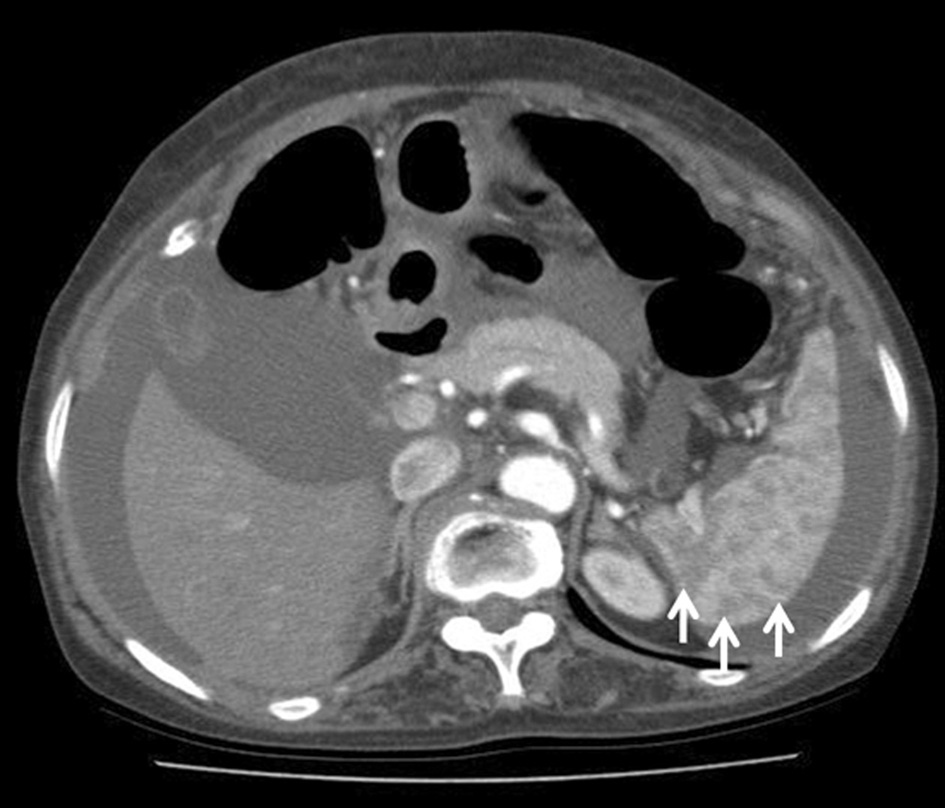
Initial abdominopelvic computed tomography reveals the spleen is occupied with multiple, well-defined, low density, lesions measured 0.5 to 1.5 cm in sized (arrows) and moderate amount of ascites.
